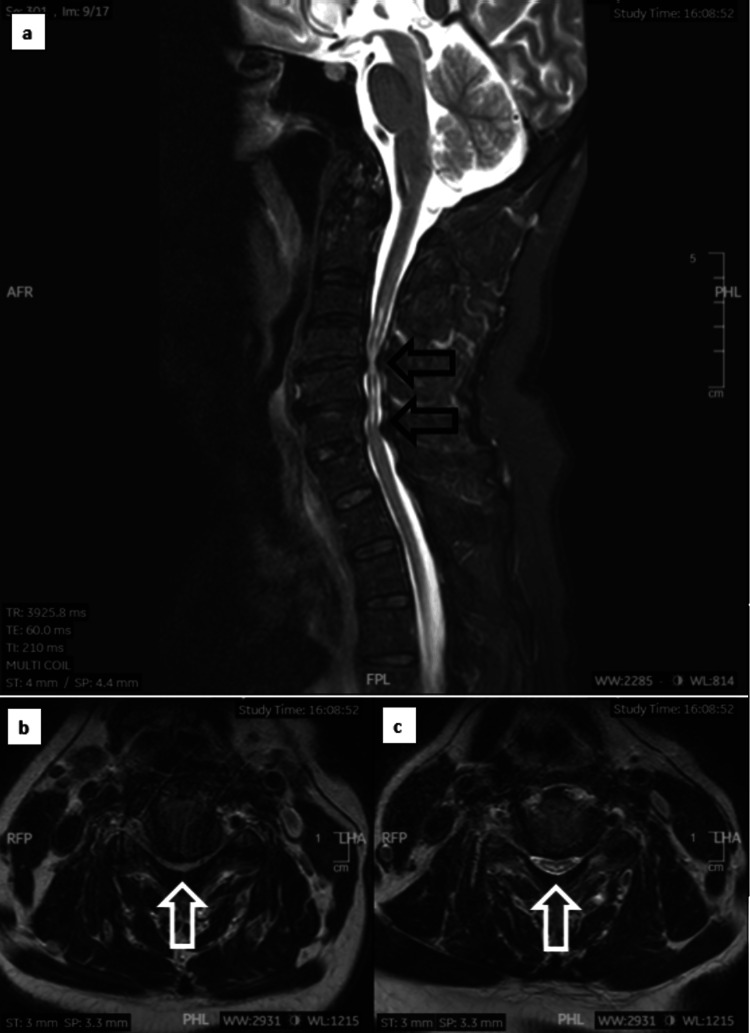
MRI of the cervical spine in various views. a) Spinal cord compression with cord changes spanning from C4 to C6, with a more severe level of compression observed at the C4/C5 level (indicated by the black arrow) (sagittal view). b) Severe central cervical canal stenosis at the C4/C5 level, characterized by complete cerebrospinal fluid effacement and evident cord compression (indicated by the white arrow) (axial view). c) Moderate central cervical canal stenosis with near-complete cerebrospinal fluid effacement and cord indentation at the C5/C6 level (indicated by the white arrow) (axial view).
